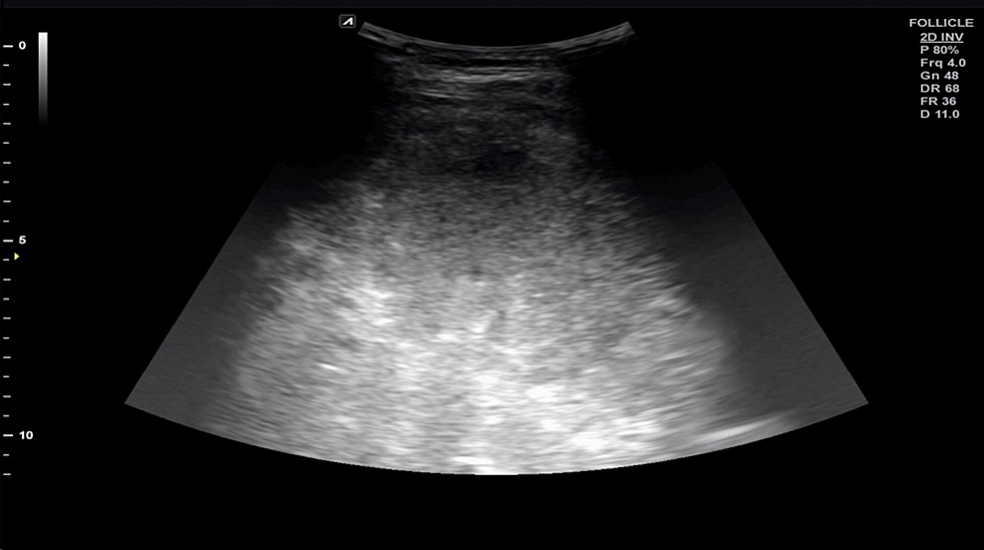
Ultrasound sonographic picture of liver section Shows an ill-defined heterogeneous hypoechoic collection in the left lobe of the liver, with free-floating echoes suggestive of internal necrosis
